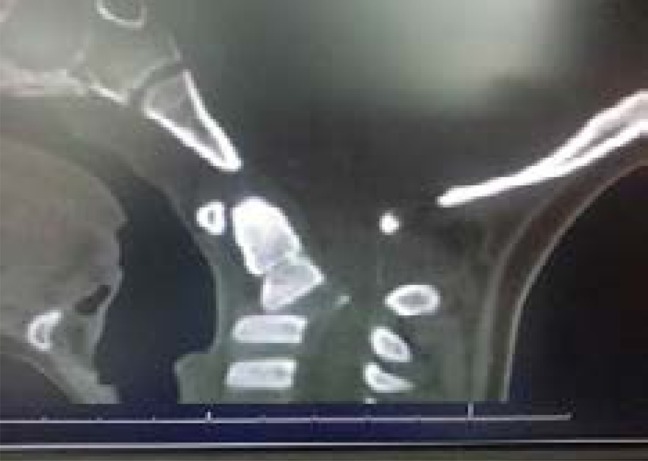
Sagittal CT scan of patient on day of accident/admission showing posterior subluxation of C2 on C3 with narrowing of the spinal canal at C2/C3 level.
